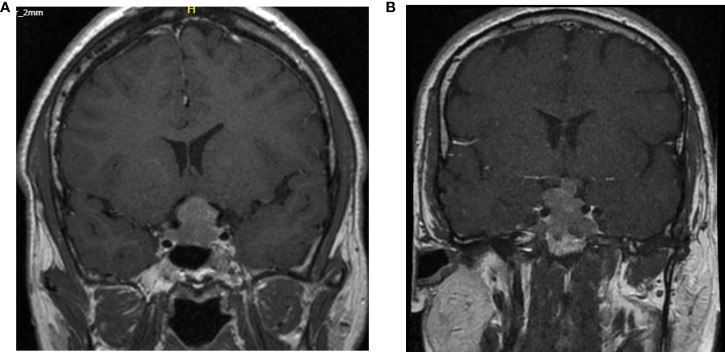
Cerebral MRI T1 weighted images with gadolinium at diagnosis of case 2 and case 5. (A) Knosp grade 4 classification of the second patient. (B) Knosp grade 3B classification of the 5^th^ patient.
